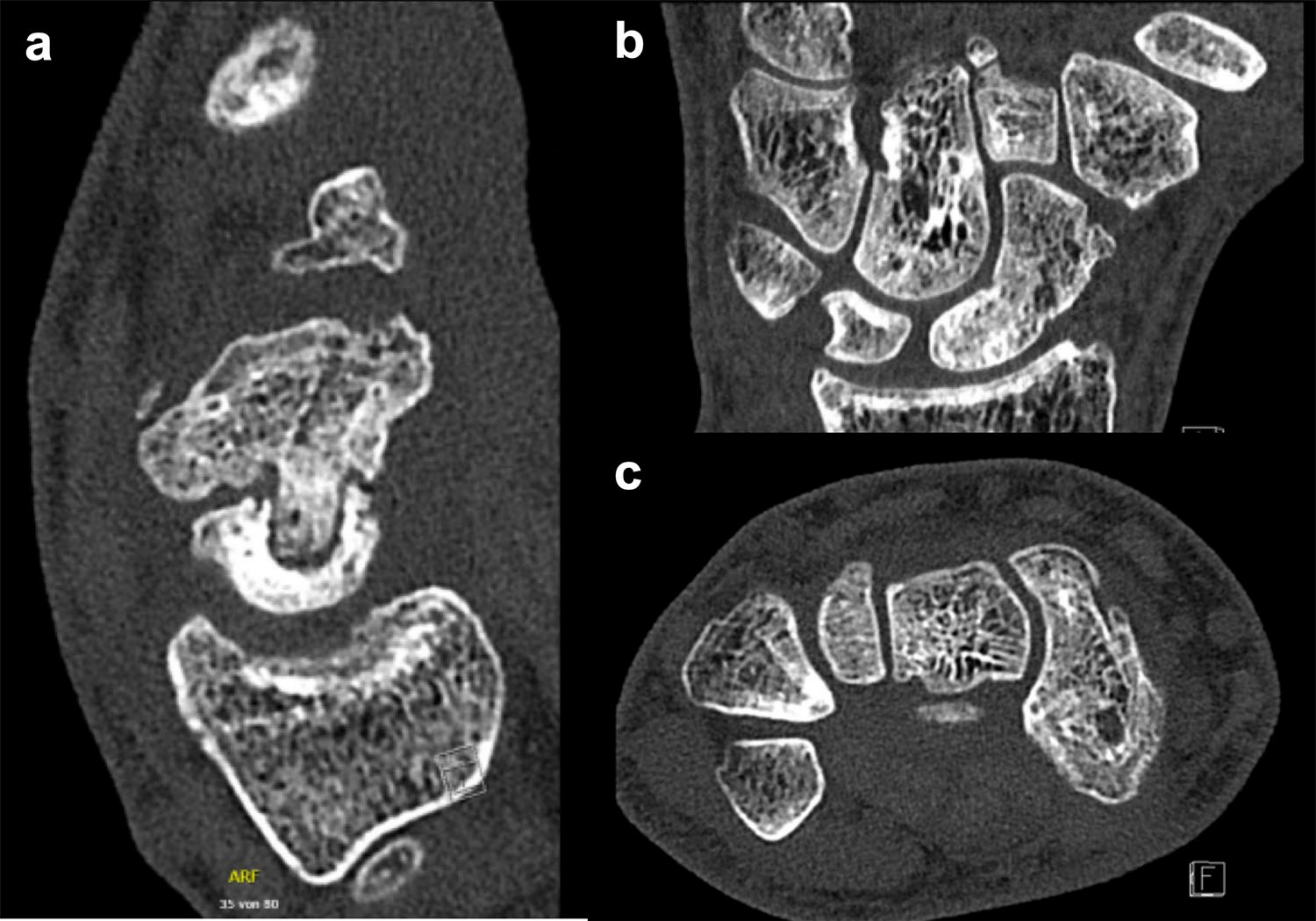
Postoperative CT scans. a Sagittal CT scans of patient #14 with failed union after 12 weeks with well notable dowel structure and integration in distal pole. b coronal and c. axial scans of patient #8 four years after successful revisional scaphoid reconstruction with dowel
